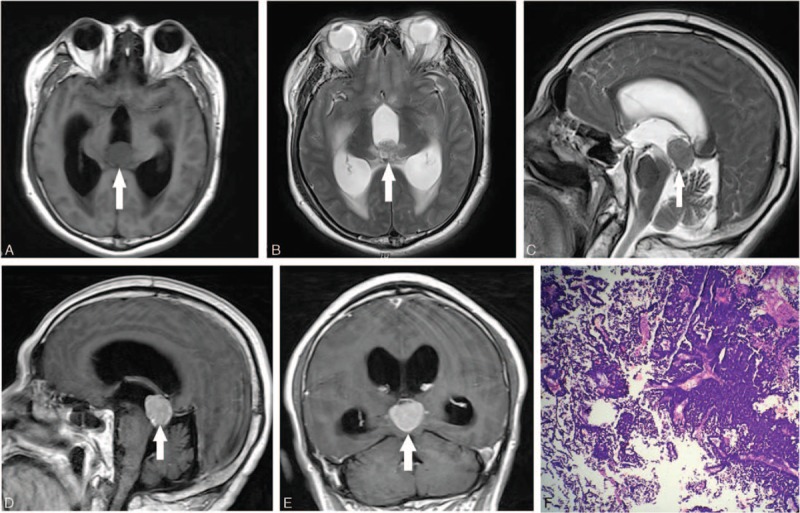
T1-weighted axial (A), T2-weighted axial (B), and T2-weighted sagittal (C) images showing the pineal region tumor (arrow). Contrast-enhanced T1-weighted images (D, E) showing the tumor (arrow) with significant enhancement. Pathological image (F) showing metastatic urothelial carcinoma with tumor cells arranged in nests.
